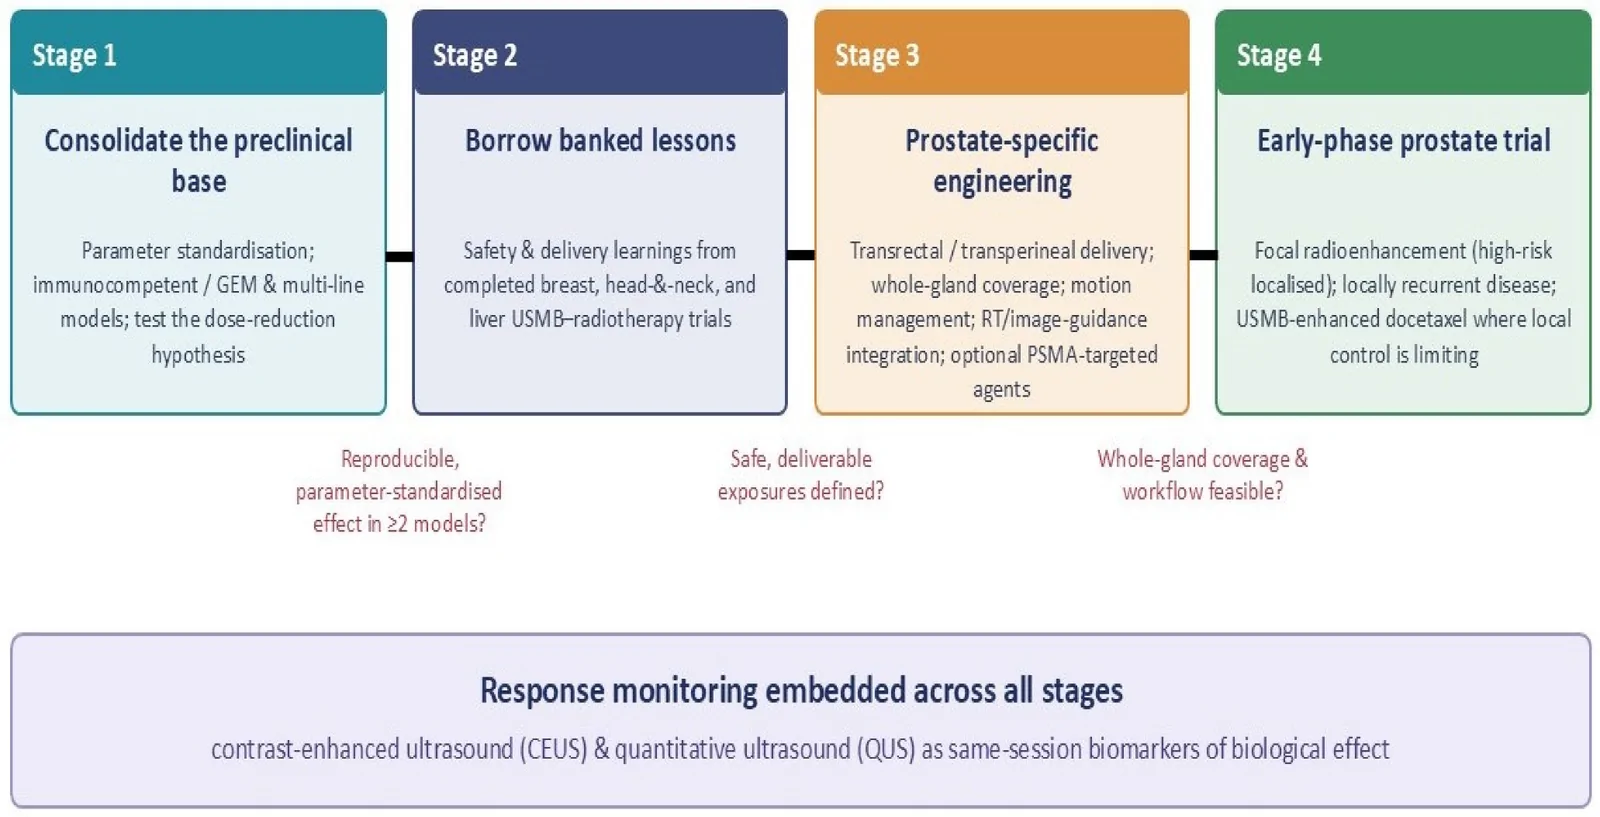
Proposed staged translational roadmap from preclinical prostate cancer models to early-phase prostate trials. Progression is gated by evidence rather than time; standardized and broadened preclinical confirmation should precede adoption of safety and delivery lessons from completed non-prostate USMB-enhanced radiotherapy studies, followed by prostate-specific delivery engineering and cautiously designed early-phase trials. Response monitoring using contrast-enhanced ultrasound (CEUS) and quantitative ultrasound (QUS) should be embedded across all stages as same-session biomarkers of biological effect. The roadmap deliberately positions first-in-prostate testing after, not before, resolution of parameter standardization and prostate-specific delivery feasibility. Author-generated conceptual roadmap.
